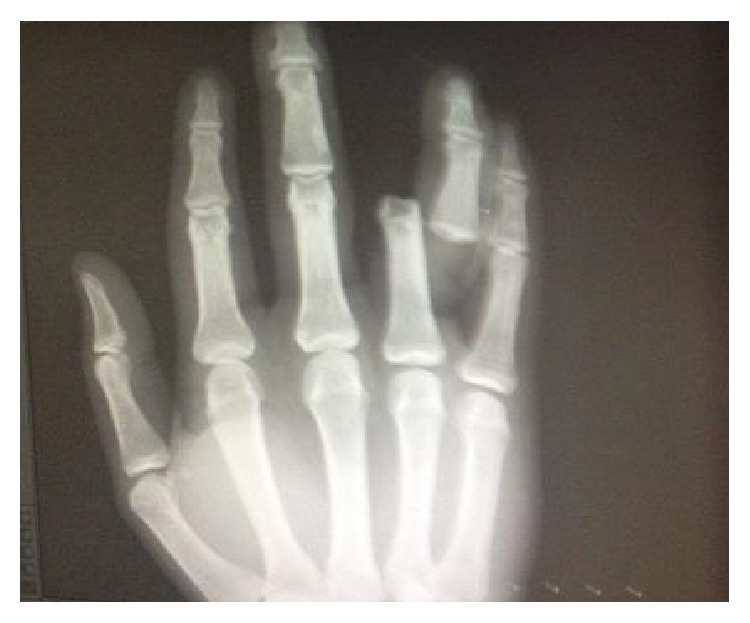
X-ray showing finger dislocation.
